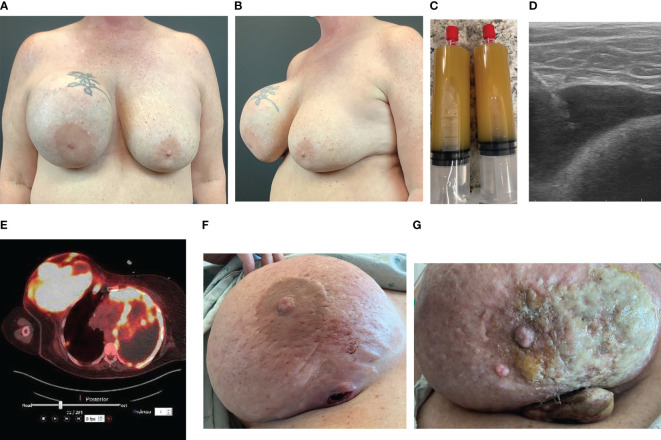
Case of BIA-SCC. (A) Anterior view of a 56-year-old female with history of macrotextured breast implant in 2006 presented with unilateral right breast swelling, pain, and capsular contracture 16 years after implantation. (B) Oblique view at presentation. (C) In-office seroma aspirate in May 2022 revealed copious amount of yellow, turbid fluid. Cytology and IHC analysis demonstrated acute inflammation and abundant squamous cells, but no malignant cells. BIA-ALCL workup negative. (D) Ultrasound shows fluid collection between implant and capsule. This patient then underwent bilateral implant removal with total capsulectomy. Intraoperative findings revealed thickened capsule with an associated tan-pink, indurated, nodular mass. Pathology revealed poorly differentiated SCC. The patient was placed on adjuvant pembrolizumab, abraxane, and carboplatin chemotherapy. (E) PET/CT 3 months post-implant removal revealed increased metabolic uptake throughout the right breast and axilla, and left chest wall and pleura, consistent with metastatic SCC. (F) By September 2022, the primary malignancy began to erode through the patient’s breast. (G) One month later the primary malignancy progressed despite chemotherapy. She expired weeks later from complications related to pleural metastases.
